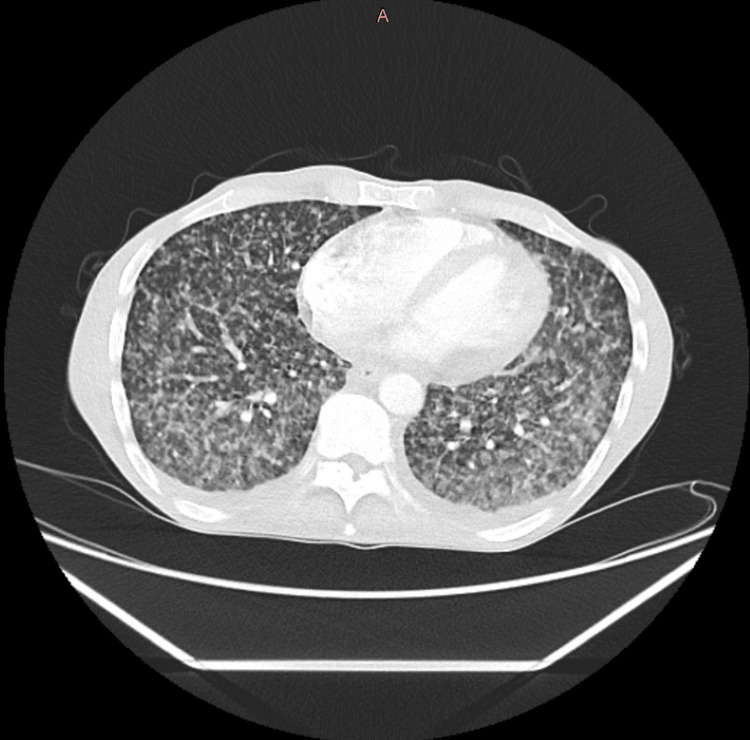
CT thorax with contrast of this patient showing disseminated tuberculosis
